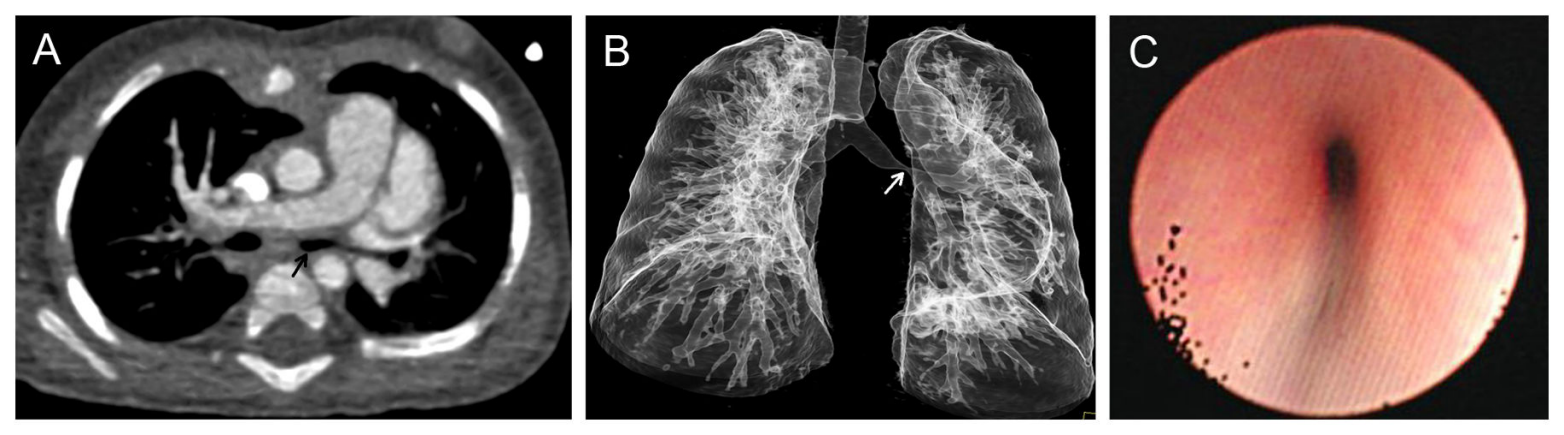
A 6-month girl with ventricular septal defect was detected by both CT and FTB with the left main bronchus compression and the local lumen became stenotic. A. CT axial image demonstrated the left main bronchus stenosis due to surrounded compression by anonymous soft tissue (black arrow). B. Volume Rendering (VR) of the tracheobronchial tree showed the local stenosis (white arrow) at the left main bronchus. C. FTB confirmed the left main bronchus stenosis, which noted extralumen pulsatile compression of the bronchus.
